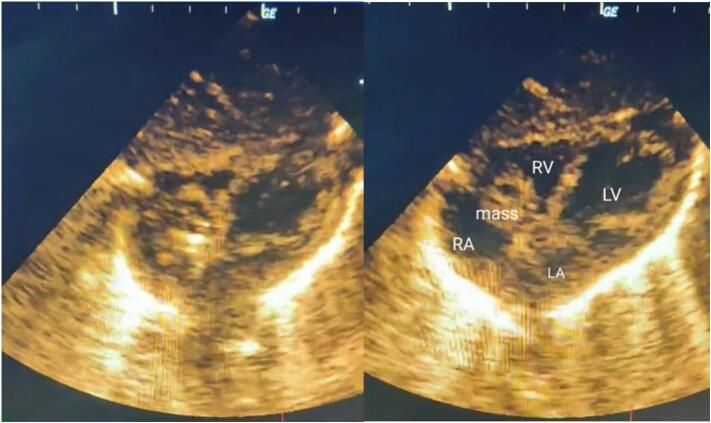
Echocardiogram shows a mass in the right atrium and prolapse of the mass across the tricuspid valve. RV: right ventricle, LV: left ventricle, RA: right atrium, LA: left atrium.
